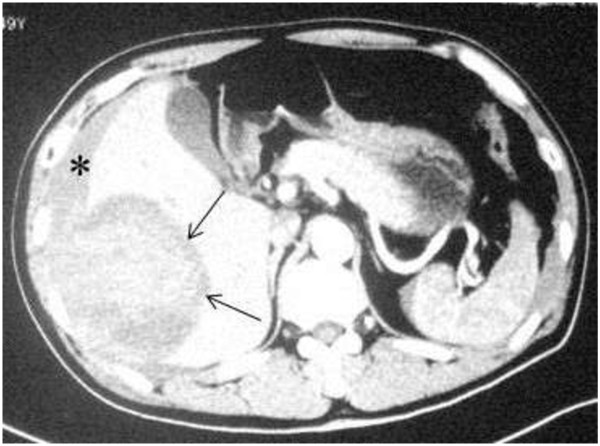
CT reveals a large tumor (arrows) in the right lobe of the liver along with an adjacent intraperitoneal hyperdense fluid collection (black asterisk).
